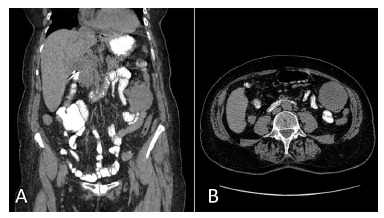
Splenic angle mass: A) CT coronal slice; B) TC axial slice (distal
